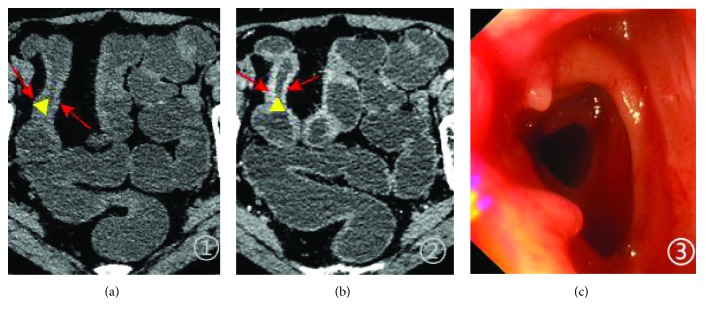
A 54-year-old woman with mild active CD, SES-CD of 4. CT plain scan shows the thickening of the bowel wall ((a) red arrowheads) and stenosis ((a) triangle) in the terminal ileum. Enhanced CT scan in the portal vein phase shows the thickened bowel wall from terminal ileum lesions which was evenly enhanced ((b) red arrowheads). The endoscopic image of the terminal ileum (c).
